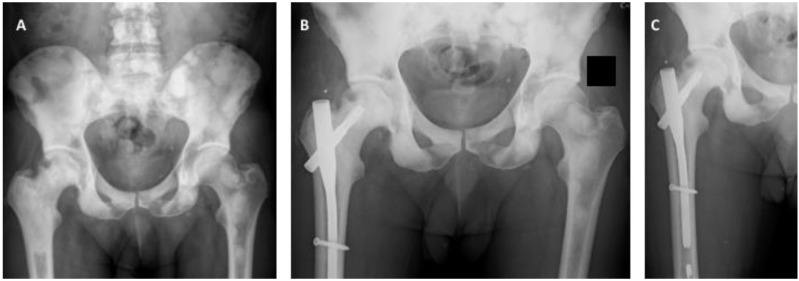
(A) Anteroposterior pelvic radiograph showing countless prostate carcinoma metastases around the lower spine, pelvis and proximal femur. Extensive invasion of the pertrochanteric area of the right proximal femur associated with mechanical pain can also be observed. (B) Postoperative anteroposterior radiograph of the pelvis after surgical stabilization of the right proximal femur metastasis using a cephalomedullary nail. (C) Anteroposterior radiograph of the right hip after surgical stabilization of prostate cancer metastasis in the proximal femur. A broken drill is visible in the medullary canal.
